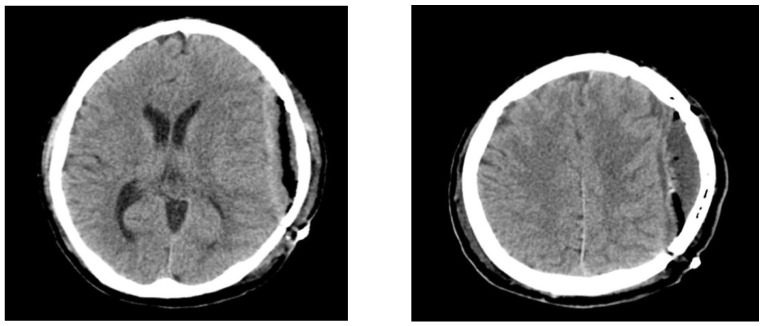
Brain computed tomography scans at 3 months after cranioplasty demonstrate air bubbles and epidural fluid collection. Although cranioplasty is a relatively simple procedure, it carries a substantial risk of postoperative infection or foreign body reaction mimicking infection [1,2] Postoperative infections are usually observed several days to months after cranioplasty operations, and delayed infections presenting 6 years after cranioplasty, as seen in our case, are rare. To the best of our knowledge, no studies have reported aphasia caused by delayed epidural abscess after cranioplasty. A 46-year-old man was admitted to our emergency room (ER) with a complaint of difficulty speaking that started 3 days prior. Left frontotemporoparietal (FTP) craniectomy and hematoma removal were performed for acute subdural hematoma 6 years prior. At 3 months after the decompressive craniectomy, cranioplasty was performed to repair the skull defect using cryopreserved autologous bone. Computed tomography (CT) at 3 months post-cranioplasty revealed postoperative air bubbles and epidural fluid collection (Figure 1).
